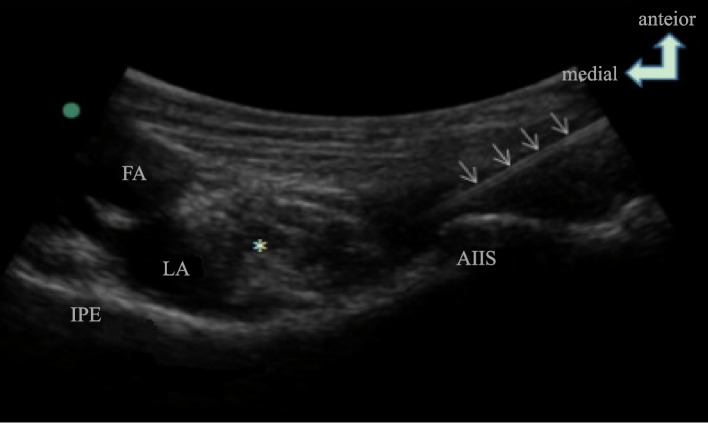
Representative image and ultrasound scan of patient receiving PENG block. Notes: White arrow is ultrasound-guide tracing of puncture needle; * Iliopsoas tendon is marked. Abbreviations: PENG, pericapsular nerve group; AIIS, anterior inferior iliac spine; IPE, iliopubic eminence; FA, femoral artery; LA, local anesthetics
